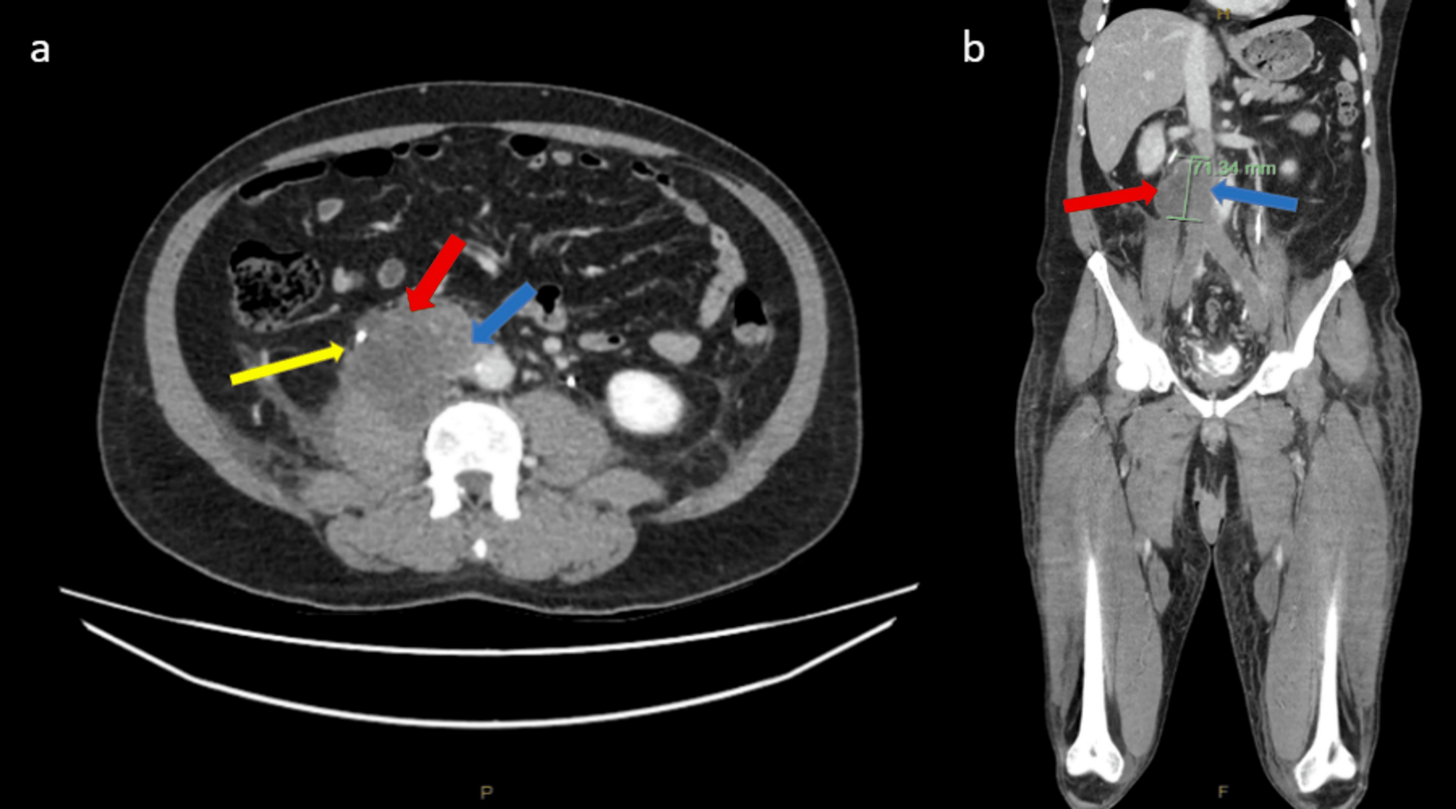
CT portal venous phase pre-operatively CT: computed tomography; IVC: inferior vena cava (a) Axial slice demonstrating an interval increase in size of the right retroperitoneal mass (red arrow) to 69 x 73 x 71 mm lying in proximity to the right ureter (yellow arrow) and IVC (blue arrow). (b) Coronal slice demonstrating the lobulated mass (red arrow) invading the IVC lumen (blue arrow)
